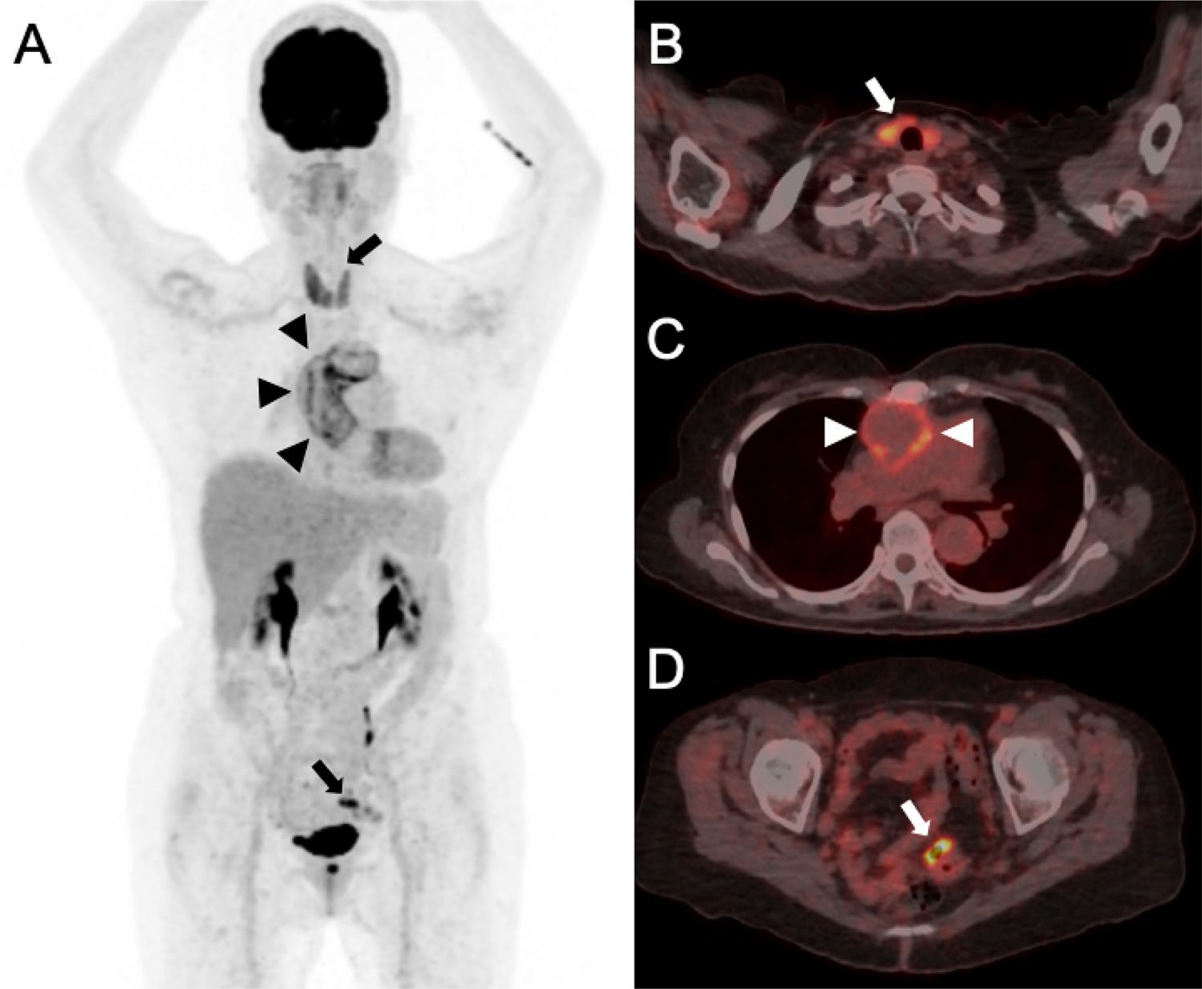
A 70-year old female patient was referred to PET/CT 14 months after the initial diagnosis of a vascular graft infection due to *Streptococcus hominis*. The reason for referral was whether antibiotic treatment could be stopped. PET/CT [maximum intensity reconstructions of PET (A) and fused PET/CT images (B–D)] showed diffuse FDG uptake along the ascendens graft (Index surgery: aortic arch replacement with 28 mm Intergard® prosthesis) (black arrow heads in A, white arrow heads in C) which was focally pronounced (A) indicating persistent infection. Antibiotic treatment was continued for another eight months and then successfully stopped (no signs for recurrence at the last control seven months later). The patient had known thyroiditis, which presented with diffuse FDG uptake (upper black arrow in A, white arrow in B); this finding was not evaluated for impact on patient management in our study, as it was already known prior to PET/CT. An unknown and relevant incidental finding was detected in the sigmoid colon with intense focal FDG uptake (lower black arrow in A, white arrow in D). This PET/CT finding was rated to have impact on patient management—subsequent coloscopy and resection revealed a colonic polyp with dysplasia.
